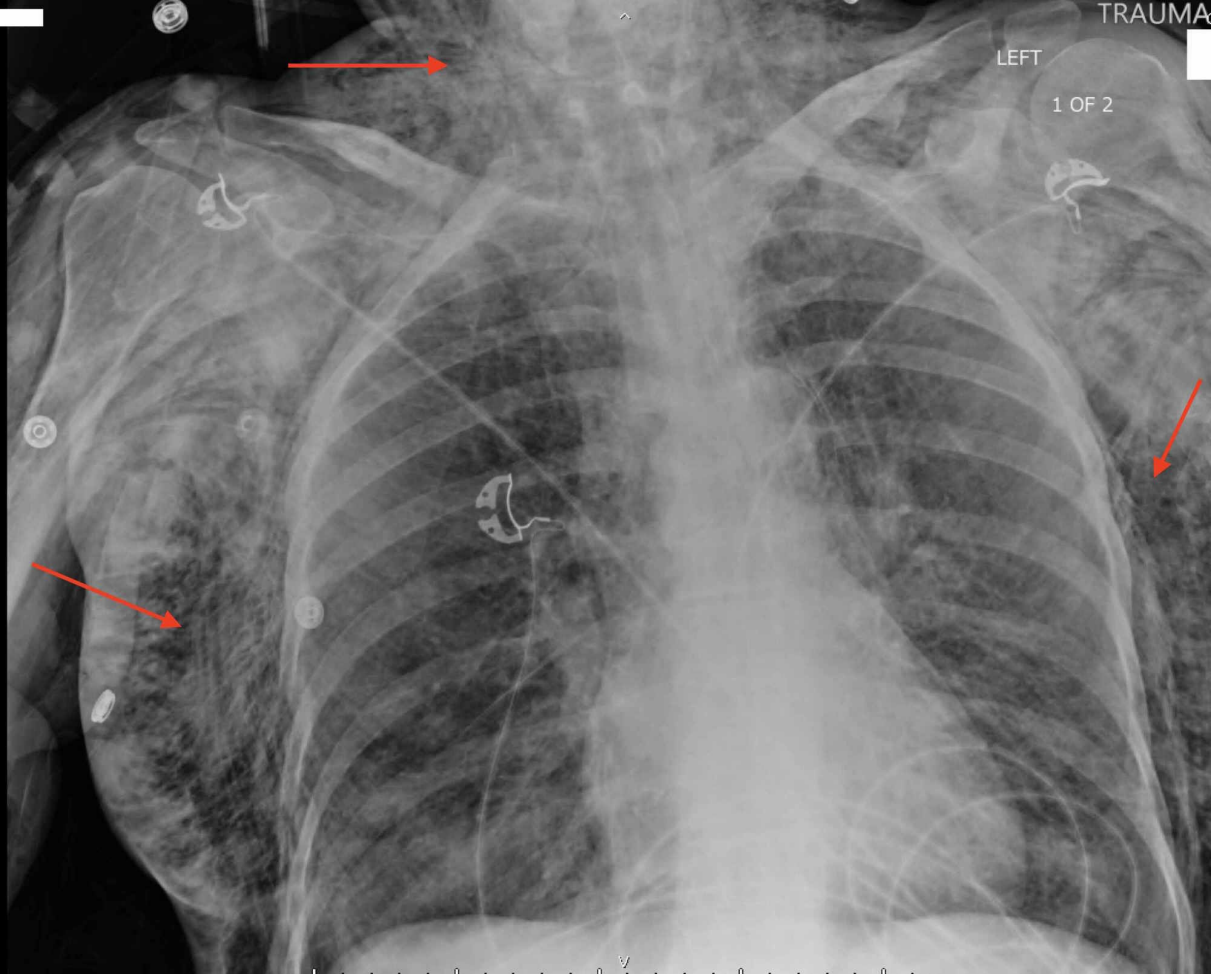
Massive Subcutaneous Emphysema Initial trauma chest radiograph showing significant widespread subcutaneous air (arrows pointing to areas with large amounts of subcutaneous emphysema).
